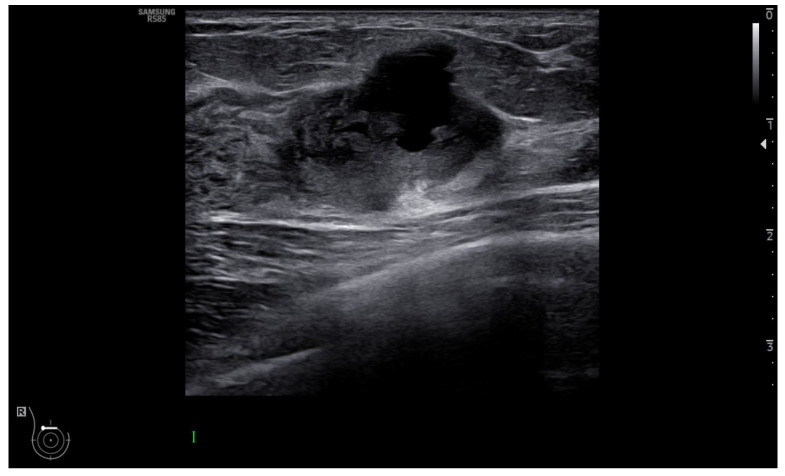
Ultrasound shows a heterogeneous hypoechoic mass lesion with small lobulations located in the upper quadrant of the left breast, highly suspicious for breast cancer.
